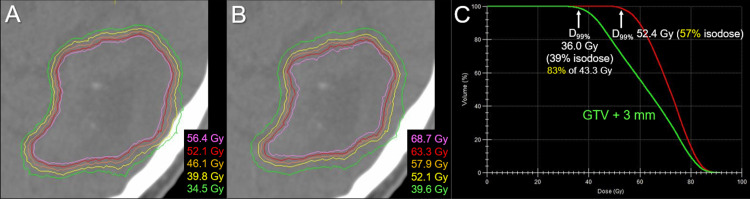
An alternative radiosurgical plan based on volumetric modulated arcs using a 5-mm leaf-width multileaf collimator The images show (A, B) the dose distributions superimposed onto an axial CT image and (C) the DVHs. (A, B) The representative isodoses are shown similarly to Figures 2A, 2B (the original plan). (A-C) The alternative plan for the initial SRS was generated using volumetric modulated arcs with the Agility^®^ collimator (Elekta AB, Stockholm, Sweden), where 52.4 Gy was assigned to the D_99%_ of the GTV as per the original plan. No constraint to the internal maximum dose of the GTV results in more concentrically laminated, steeper dose increase inside the GTV boundary (more inhomogeneous GTV dose) and more precipitous dose falloff (gradient) outside the GTV: the D_99%_ of the GTV + 3 mm (36.0 Gy) is reduced by 17% compared to that of the original plan (43.3 Gy). The cumulative BED_2_s of the GTV + 3 mm D_99%_ in the original and alternative plans are 157.1 Gy and 130.5 Gy (17% reduction), respectively. Thus, the alternative plan can attenuate the risk of relevant adverse radiation effects and provide room for GTV dose escalation without increasing the normal tissue dose outside the GTV. D_99%_: a minimum dose encompassing at least 99% of the target volume; GTV: gross tumor volume; GTV + 3 mm: GTV plus a uniform 3-mm margin; CT: computed tomography; DVH: dose-volume histogram; BED_10/2_: a biologically effective dose based on the linear-quadratic formula with an alpha/beta ratio of 10/2
